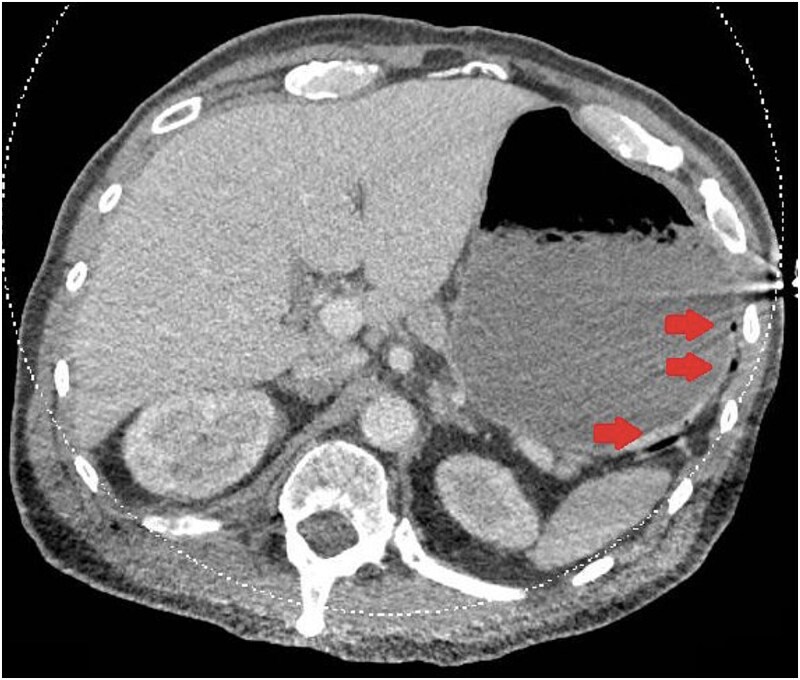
Axial CT image of the abdomen demonstrating gastric pneumatosis, indicated by red arrows.
